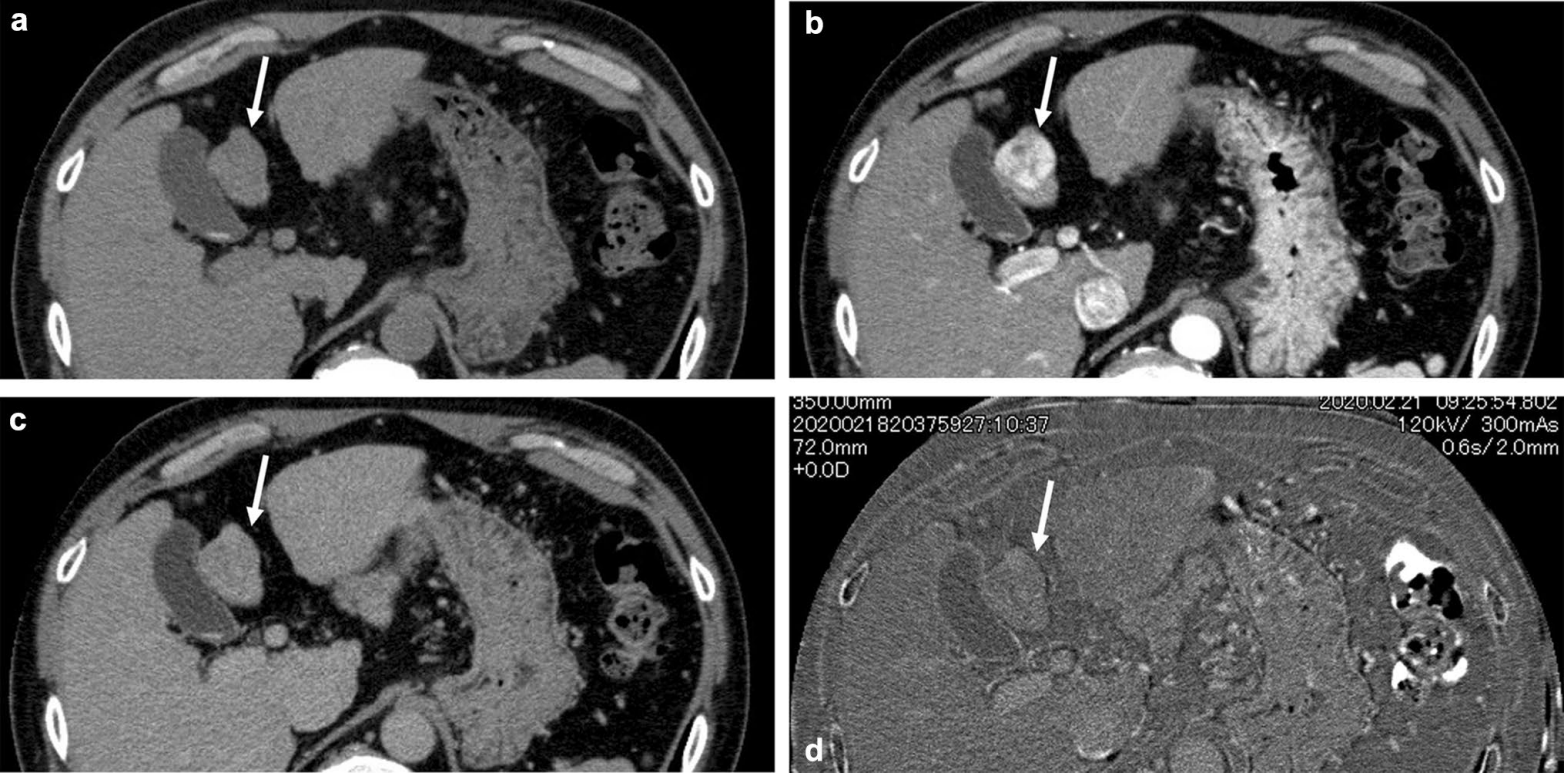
57-year-old man with chronic hepatitis B infection and habitual alcohol over-intake. Pathologically, a well differentiated hepatocellular carcinoma, mainly consisting of compact component, associated with fibrous capsule, was diagnosed. Within the tumor, apparent septal formation was seen, but no fatty change was evident. a Precontrast CT. The tumor exhibits almost iso- to minimally lower density than the background liver (arrow). Measured densities were 50 and 54 Hounsfield units for the tumor and the background liver (segment 4), respectively. b Arterial phase CT. The tumor shows heterogeneous enhancement (arrow). c Equilibrium phase CT. The washout of the tumor is not apparent, showing heterogeneous appearance (arrow). d Neither on extracellular volume fraction (ECV) map, the tumor is hard to be recognized. Measured ECV were 33.5 and 26.8% for the tumor and the background liver, respectively. In this case, although the precontrast density of the tumor or the liver were almost identical, it was considered higher ECV of the tumor obscured the apparent washout
